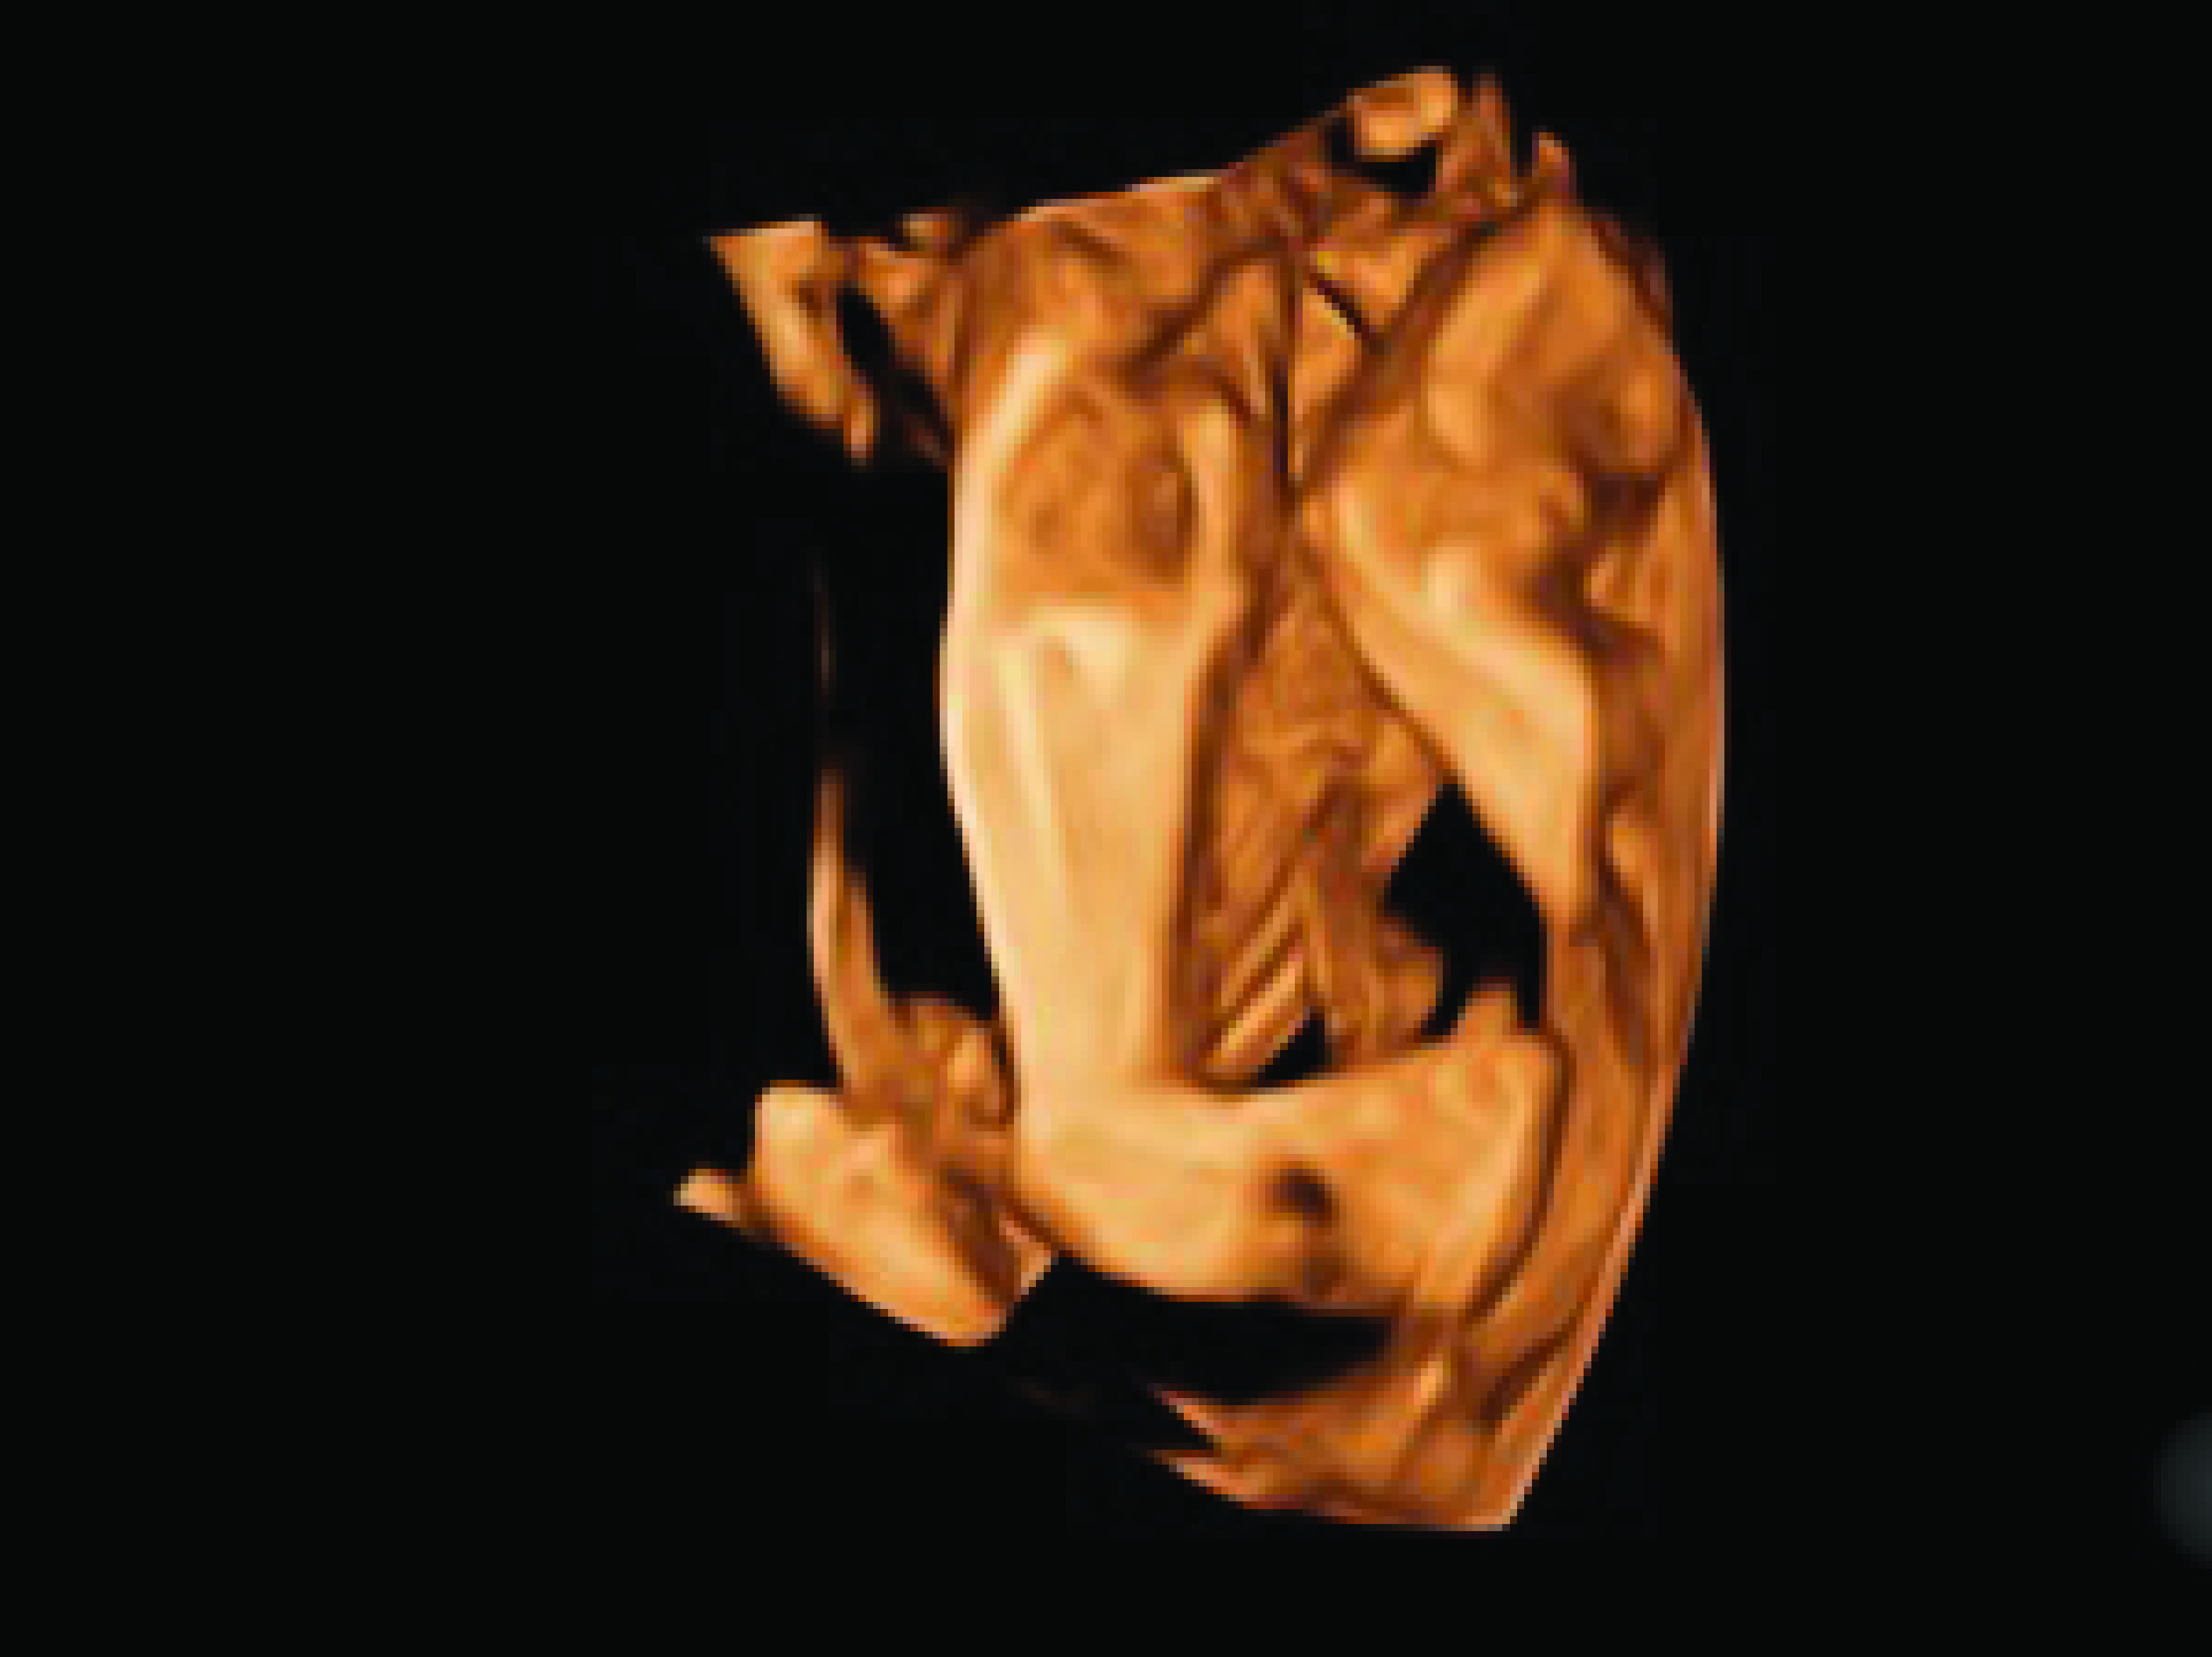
Sonographic image of clubfoot.
